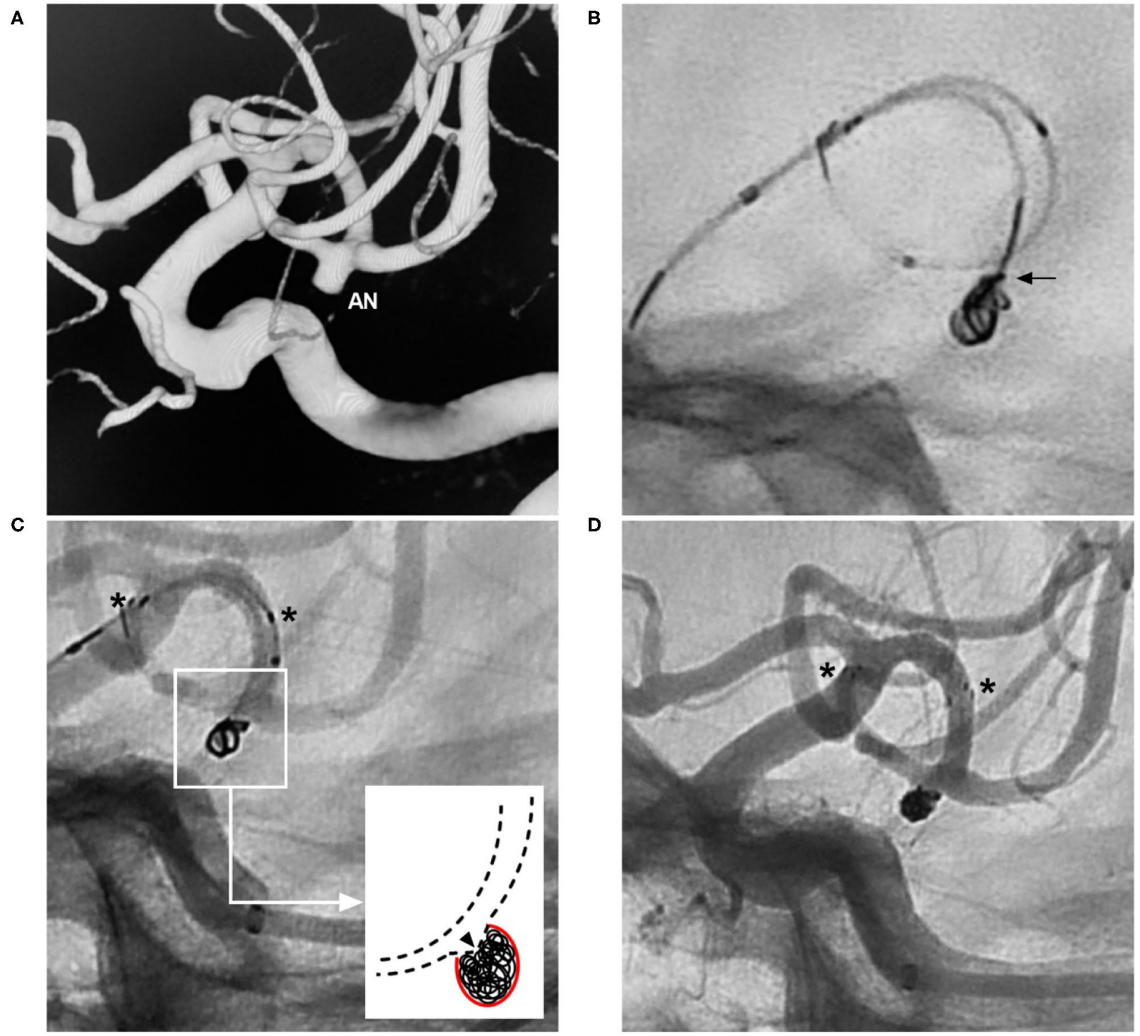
Herniation technique with the NAS. (A) Three-dimensional DSA of the ICA showing an MCA aneurysm (AN). (B) X-ray film showing protrusion of the coil into the parent artery (arrow). (C) Unsubtracted DSA showing that after NAS deployment, the coil was pushed into the aneurysm sac by the herniation technique; the asterisks show the proximal and distal markers of the NAS, and the magnified inset (long arrow from the frame) shows herniation of the struts (triangle) of the NAS into the aneurysm. (D) Postoperative DSA of the ICA showing Raymond-Roy class 1 embolization of the aneurysm; the asterisks show the proximal and distal markers of the NAS. AN, aneurysm; DSA, digital subtraction angiography; ICA, internal carotid artery; MCA, middle cerebral artery; NAS, Neuroform Atlas stent.
